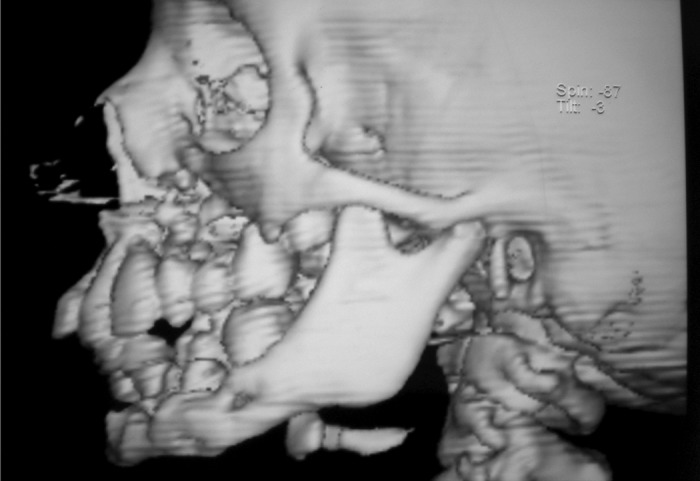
3D reconstructive view left side of CT-face
